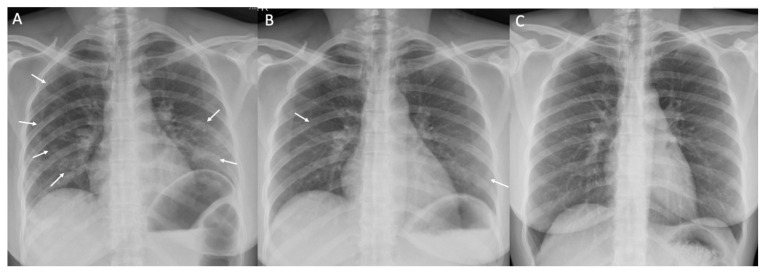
Chest X-rays during the hospitel course determined by Rama Co-RADS in one representative patient. A 43-year-old woman with a body mass index of 24 kg/m^2^ presented with cough and dyspnea for 1 day (13 days after contact). (A) Initial chest X-ray obtained on admission (day 2 of illness) shows multifocal poorly defined patchy opacities and/or consolidation (arrows) in both lungs, compatible with COVID-19 pneumonia (Rama Co-RADS category 5). (B) Follow-up chest X-ray obtained on day 6 of illness and after 4 days of favipiravir and corticosteroid treatment shows a marked improvement with faint residual opacities (arrows) in some affected areas. (C) Follow-up chest X-ray obtained on day 13 of illness shows complete resolution of COVID-19 pneumonia.
